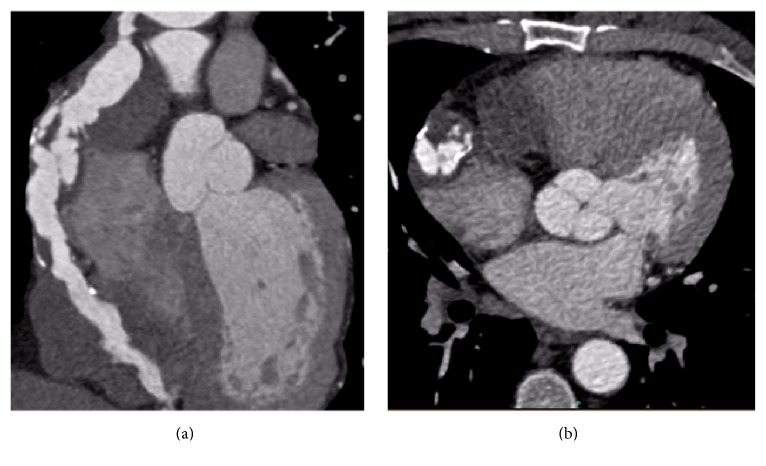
Coronal and axial slices from CT coronary angiogram with the calcified aneurysmal SVG to PDA graft.
